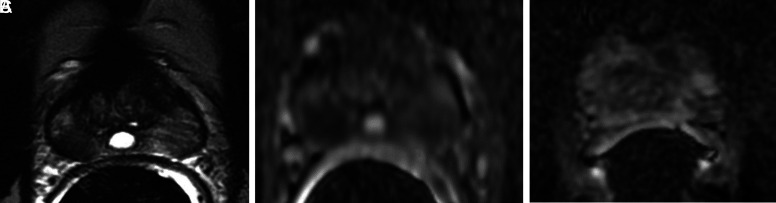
Magnetic resonance images of axial sequences T2WI (A), ADC (B), and DWI (C) of large tumor contact. The prostate gland shows a tumor extension with large contact: in the right peripheral postero-lateral portion of the prostate gland, there is a hypointense lesion in T2WI with wide contact with the capsule without irregularities thereof or without signs of macroscopic extracapsular extension. The lesion is all contained within the capsule and can be classified into a T2 stage. ADC, apparent diffusion coefficient; DWI, diffusion-weighted imaging.
